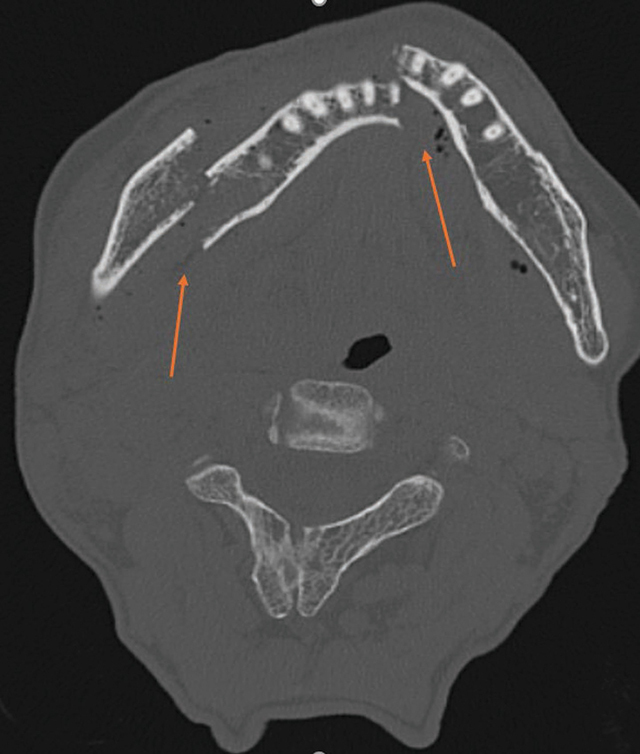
Axial CT scan showing a bifocal mandibular fracture with displacement.
